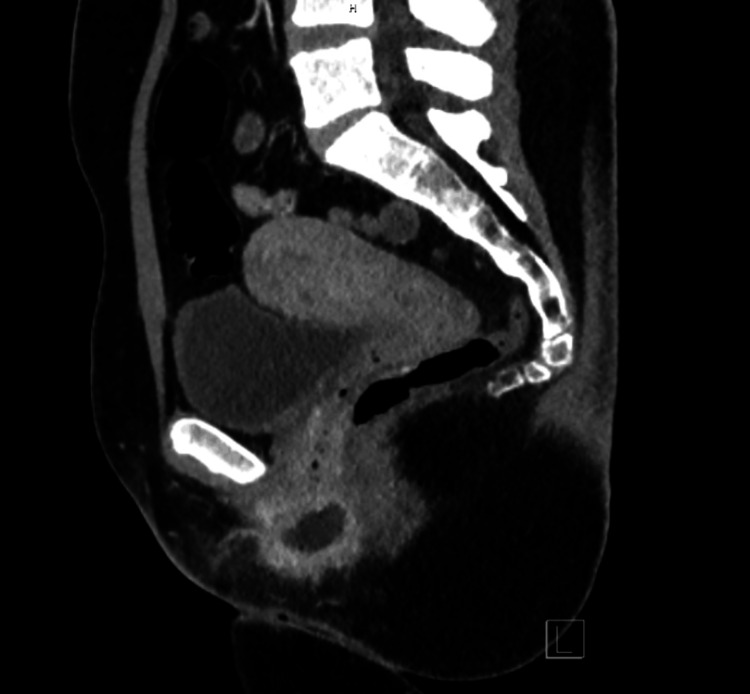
Sagittal image of abdominopelvic CT Showing low-density fluid tracking posteriorly and superiorly from a Bartholin's abscess, along the right side of the distal rectum and anal verge.
